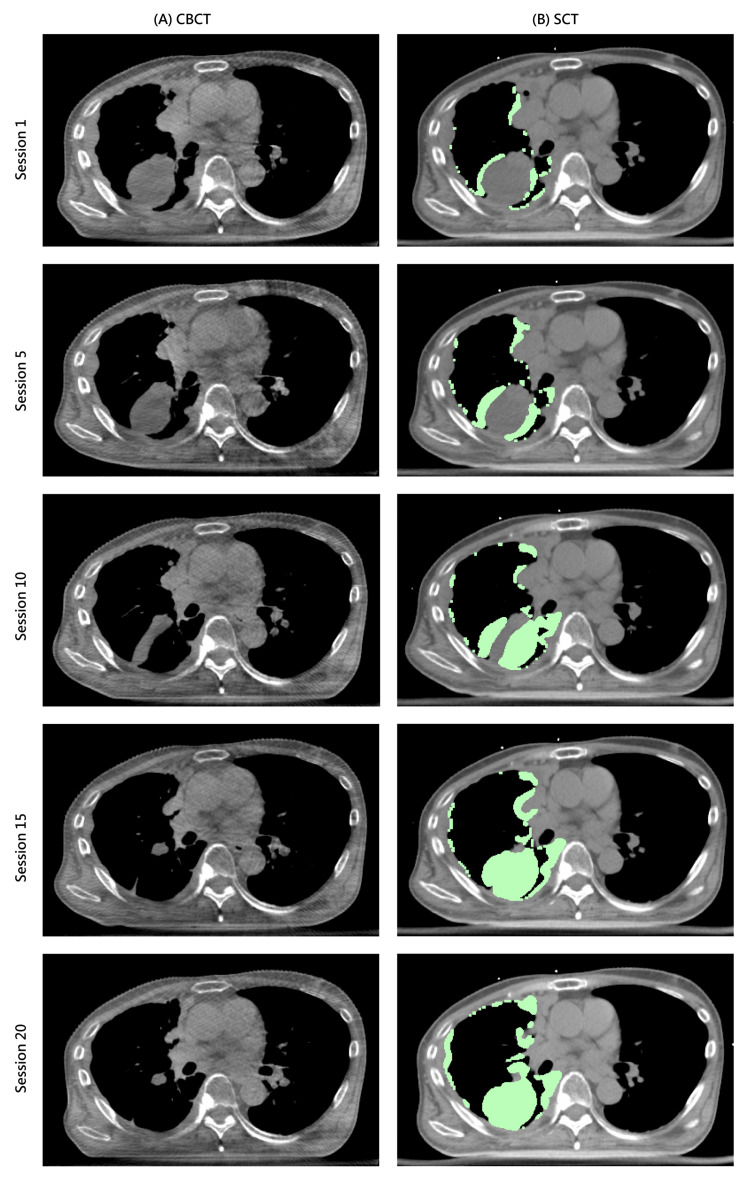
The (A) CBCT and (B) sCT of the 1st, 5th, 10th, 15th and 20th RT session. The overridden area is shown in light green. CBCT: cone-beam computed tomography; sCT: synthetic computed tomography.
